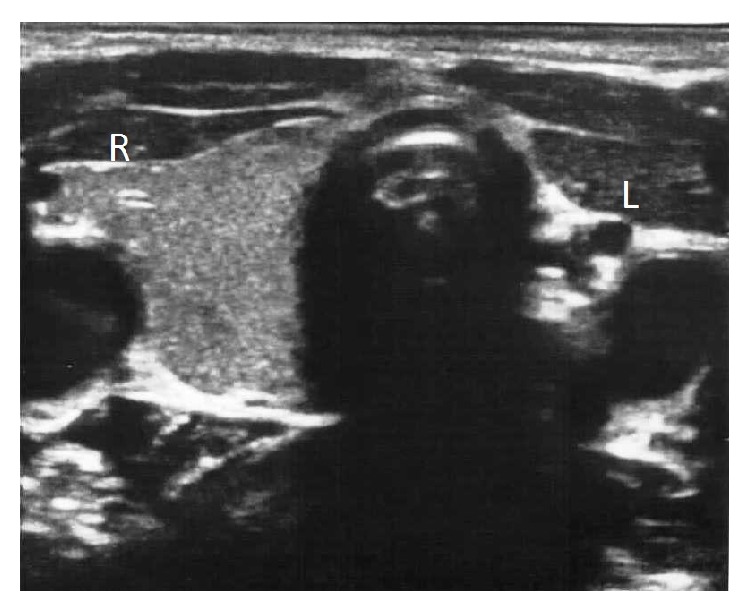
On transverse sonogram of the thyroid, right lobe (R) displays normal, homogeneous echotexture without any nodule. Left lobe cannot be demonstrated. Strap muscles and vascular structures are displaced to fill the location of the left lobe (L).
